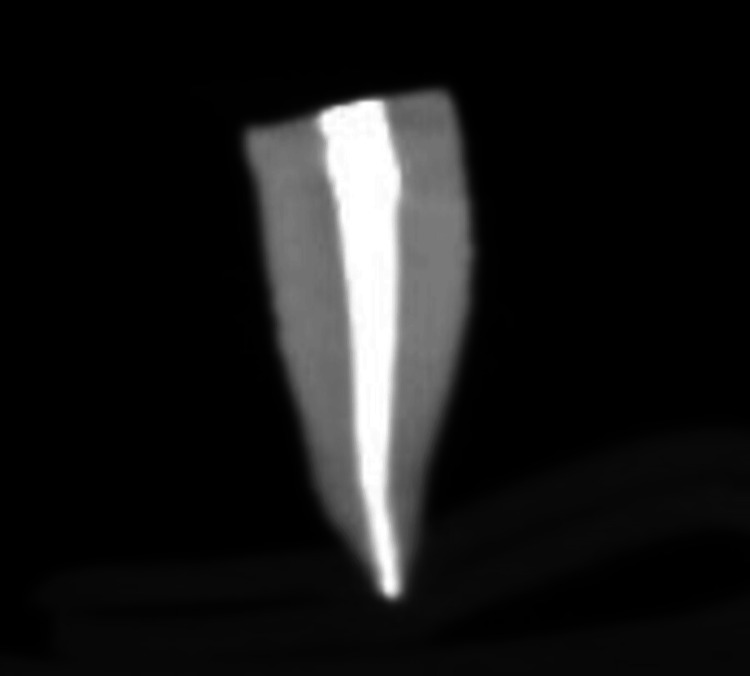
CBCT image after obturating with AH Plus sealer The white continuous shade (radio-opacity) at the center of the tooth shows the obturating material (gutta-percha along with AH Plus sealer). CBCT: cone-beam computed tomography
